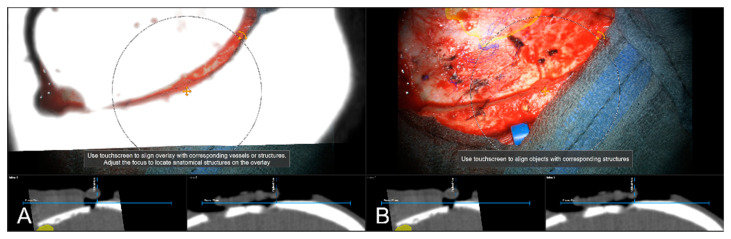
Using an intraoperatively conducted CT scan for automatic patient registration after craniotomy for evaluation of navigation accuracy showing a sufficient in-plane match of MIP (A) and patient anatomy (B). (In parallel view of MIP projection and patient anatomy (upper part) and inline views with the recent focus plane (blue line) and the optical axis (dashed blue line) in the bottom part).
